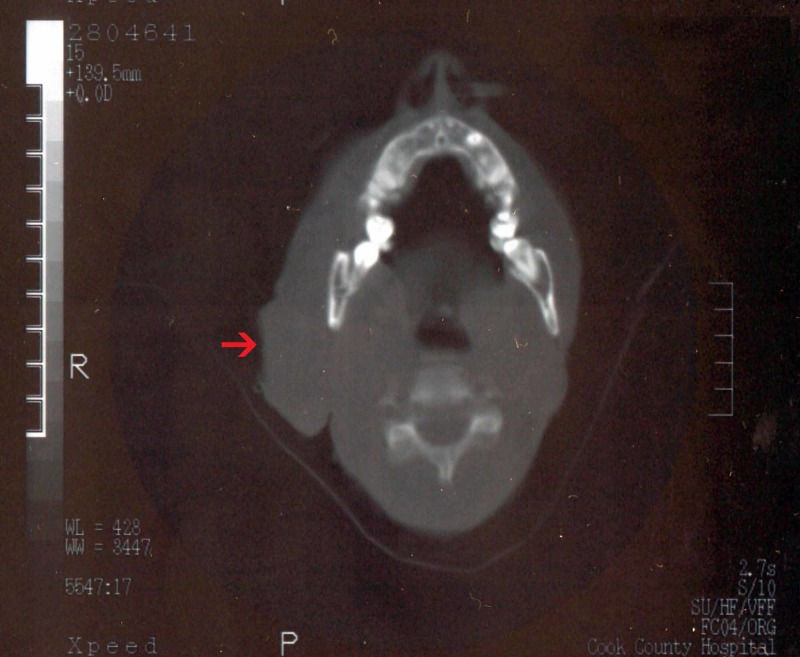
CT scan of the neck showing right-sided neck mass (red arrow).
